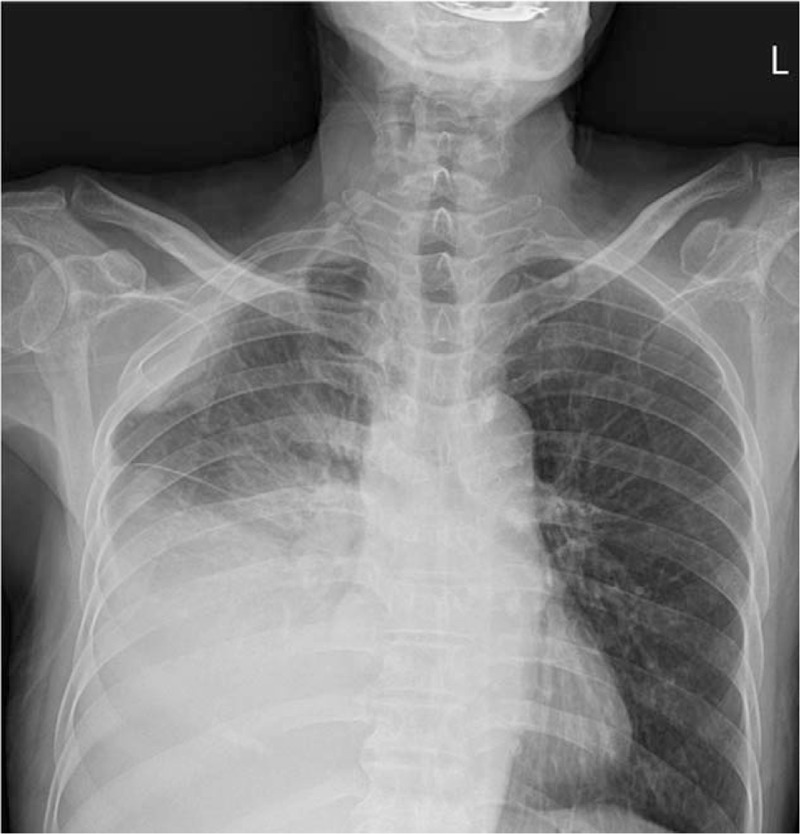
Chest X-ray revealed pleural effusion at the right side with pleural based nodular opacity.
